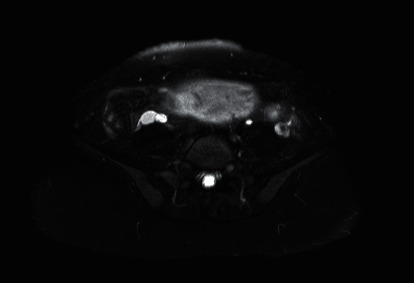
MRI of the abdomen depicting 12 mm uncomplicated appendicitis with surrounding inflammatory changes but no evidence of perforation.
